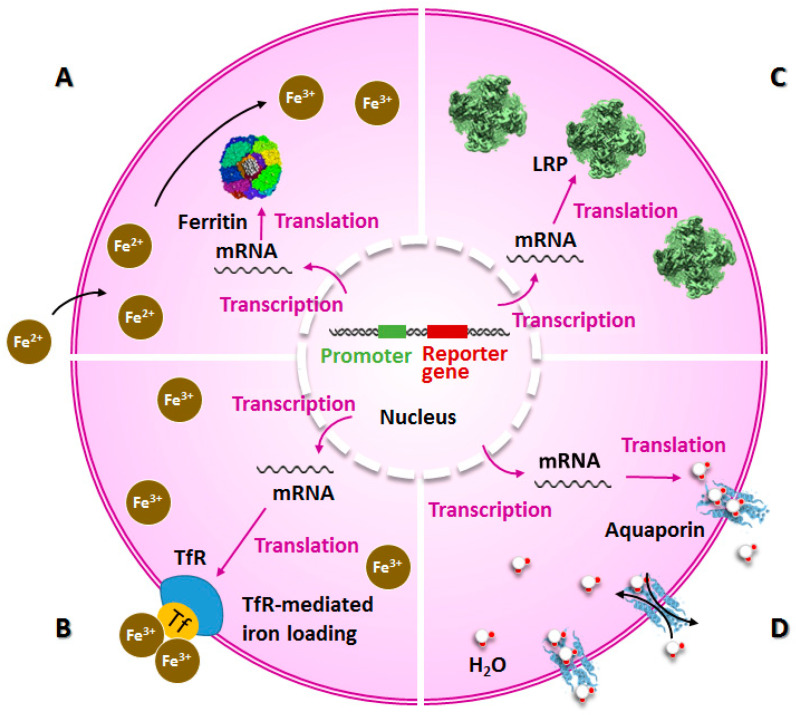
The principle of MRI reporter genes commonly used in brain imaging. (A) The expression of ferritin will cause intracellular iron particles to aggregate, resulting in a decrease in the T2 signal in MRI images. (B) The expression of TfR increases the iron uptake of cells and shows low signal intensity on T2 images of MRI. (C) LRP has a high chemical exchange rate and can be used in CEST MRI. (D) Aquaporin can increase water diffusivity and produce contrast in diffusion-weighted MRI.
